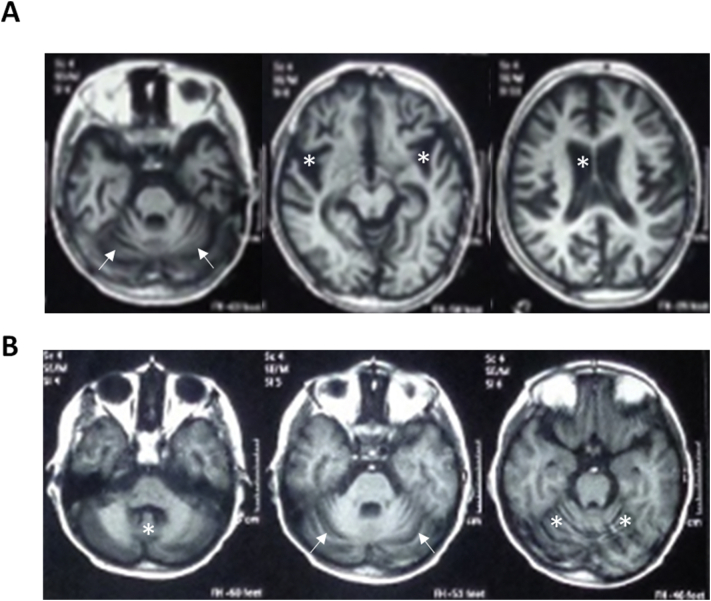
Neuroimaging findings. (A) Family A (patient 2), axial T1-weighted MRI sections show diffuse cerebral and cerebellar atrophy (white arrows), with secondary enlargement of the lateral ventricles, sylvian scissure, and subarachnoid spaces (asterisks). (B) Family B, axial T1-weighted scans showing cerebellar atrophy (white arrows) with cortical thinning and fissure enlargement of the vermis and hemispheres (asterisks).
